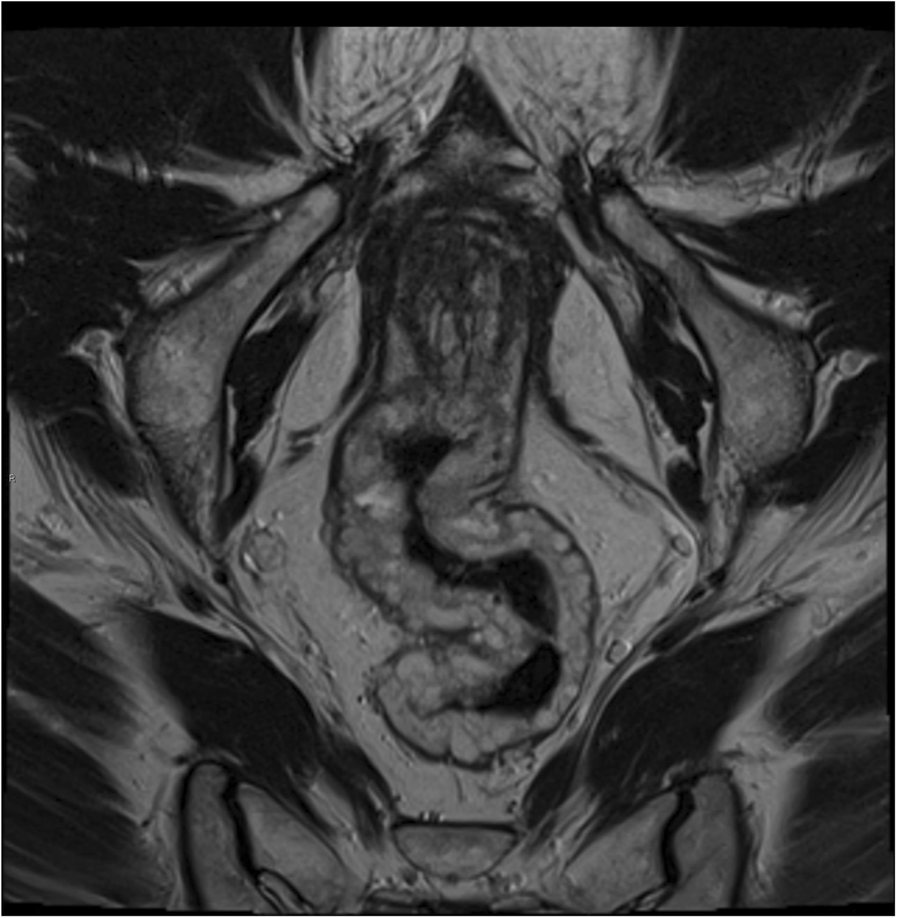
Coronal T2 weighted MRI pelvis. Nodular, predominantly high signal thickening of rectal wall
